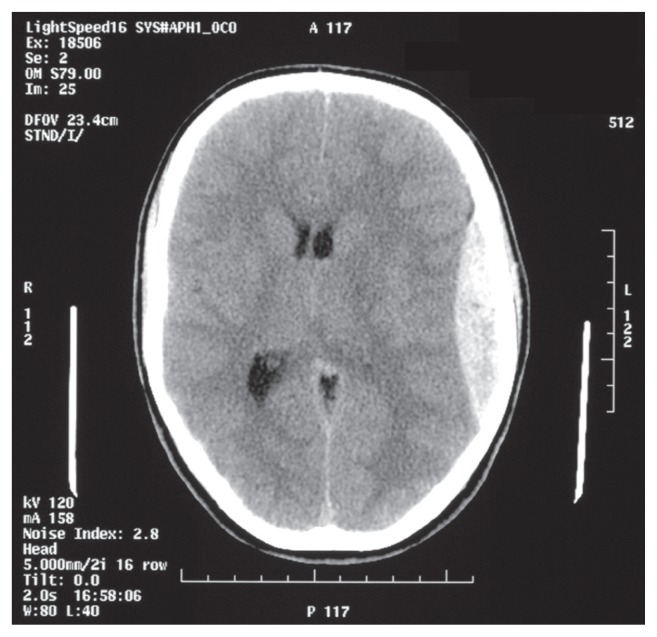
CT scan demonstrating left extradural haematoma.
